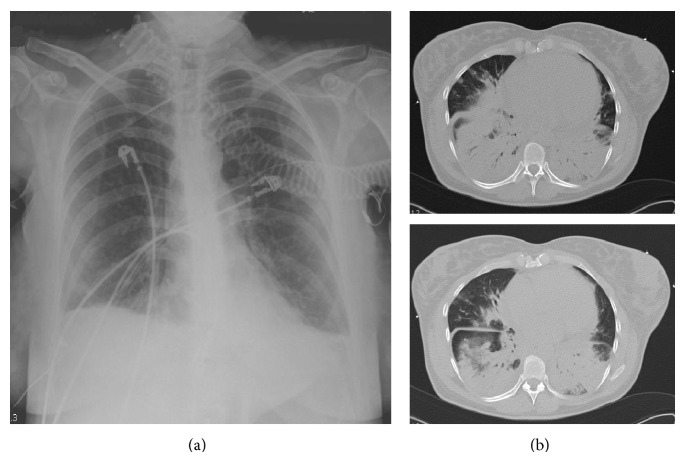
Posteroanterior lung radiograph of the mother described in Case 1 on the day of delivery (a) and computed tomography of the thorax on the day after delivery (b).
